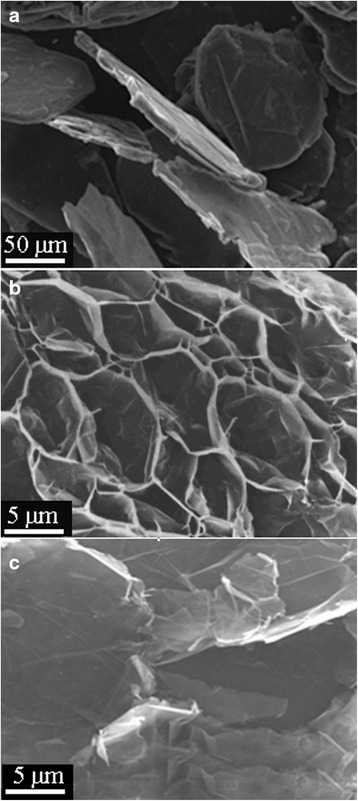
SEM images. a Natural disperse graphite. b TEG. c TEG after 3 h of ultrasonic dispersing in acetone (GNPs)
